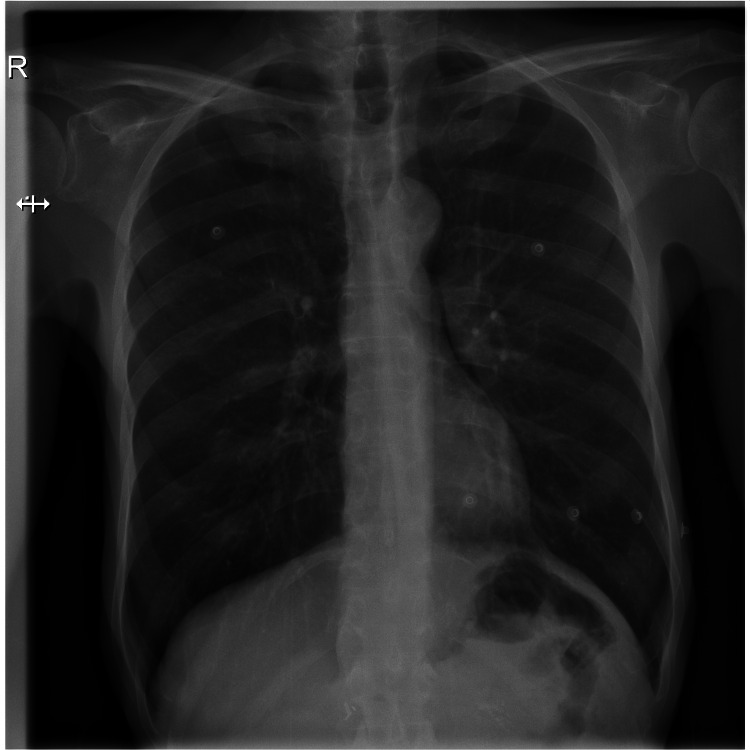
Chest X-ray showing a small heart structure.
